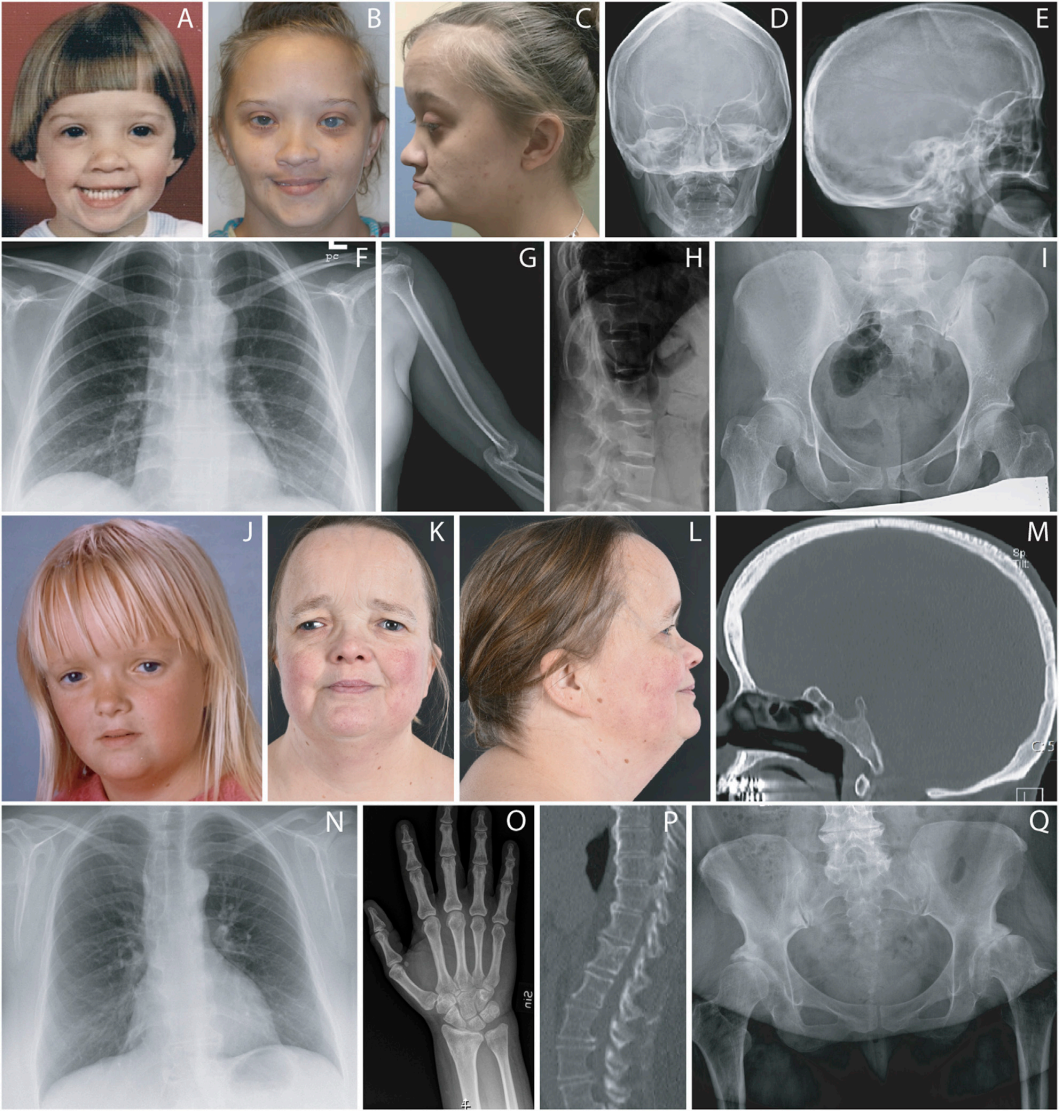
Clinical and radiographic findings at different ages (A–C): Facial features of patient 1 at 4.5 and 30 years. Note broad and prominent forehead, sparse anterior hairline and medial eyebrows, prominent eyes, epicanthal folds, hypertelorism, telecanthus, depressed nasal root, bulbous nasal tip, prognathism, malar flattening, thin upper lip vermilion and mildly posteriorly rotated ears. (D–I): Radiographic examination of patient 1 showed mild dolichocephaly with frontal bossing, dolichospondyly, a mildly narrow thorax, gracile long bones, and flat capital femoral epiphyses. The elbow joint was dislocated. (J–L): Facial features of patient 2 at 7 and 49 years. Note hypertelorism with telecanthus, down slanting palpebral fissures, depressed nasal bridge, maxillary hypoplasia, broad and prominent forehead, mildly posteriorly rotated ears and short neck. The photographs are published with informed signed consents from both patients. (M–Q): Radiographic examination of patient 2 showed dolichocephaly with frontal bossing, dolichospondyly, a narrow thorax with glenoid dysplasia, gracile ulna and radius and hip dysplasia. The computer tomography of the lumbar spine radiograms showed spondylosis and spinal canal stenosis with posterior scalloping of the vertebral bodies. The radiograms of the hip joints showed advanced degenerative joint disease along with subluxation of the left. The femoral heads were small, and the femoral necks were short.
